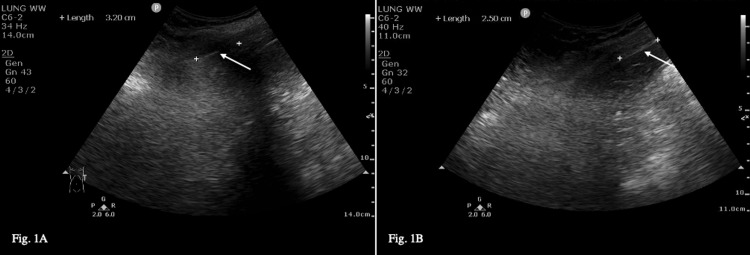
Pre-block (A) and post-block (B) diaphragm ultrasound for case 1.
